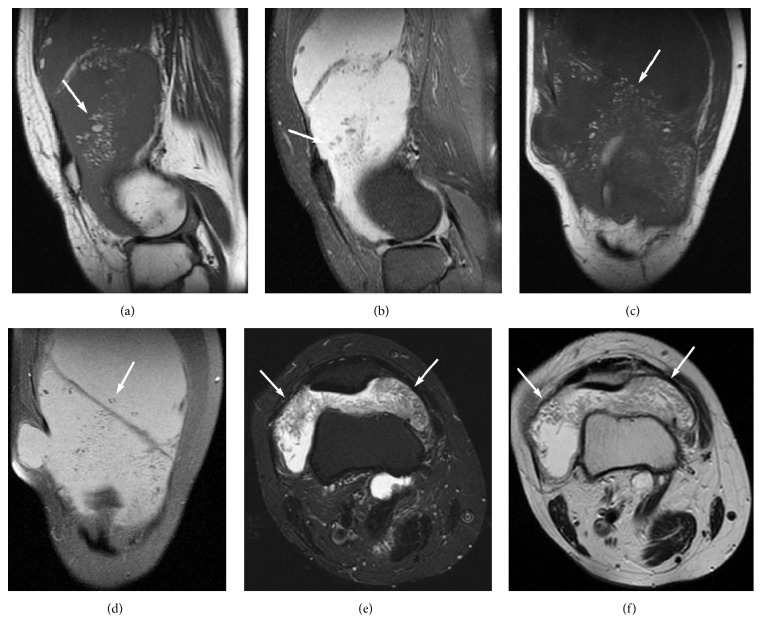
MR images on sagittal T1-weighted (a) and PD-weighted with FS (b), on coronal T1-weighted (c) and PD-weighted with FS (d), and on axial STIR (e) and T2-weighted (f) demonstrate synovial proliferation and a large primarily fat-signal mass on all MR sequences, filling much of the suprapatellar bursa with a frond-like appearance, which is surrounded by excessive joint fluid. The innumerable individual villi are small in this case, with small quantities of interposed fluid, creating a feathery appearance (arrows). These findings are indicative of a large feathery-appearing LA filling the suprapatellar recess.
